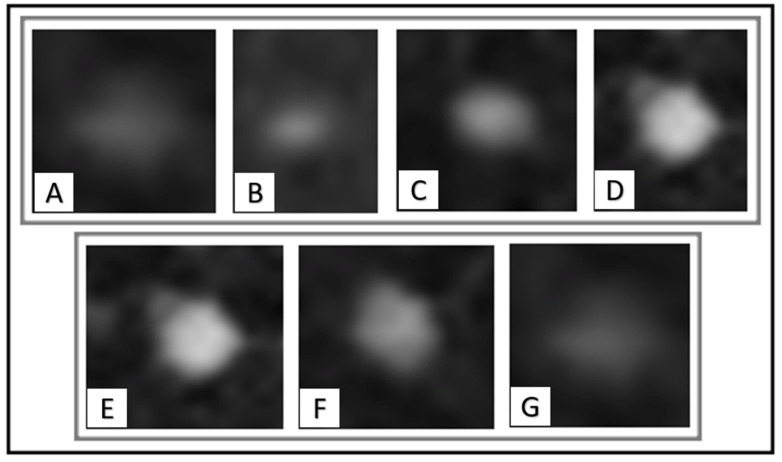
Examples of lung nodule contrast categorization ((A): very low contrast; (B): low contrast; (C): moderate contrast; (D): high contrast) and density categorization ((E): solid; (F): part-solid; (G): pure ground glass) at CT.
